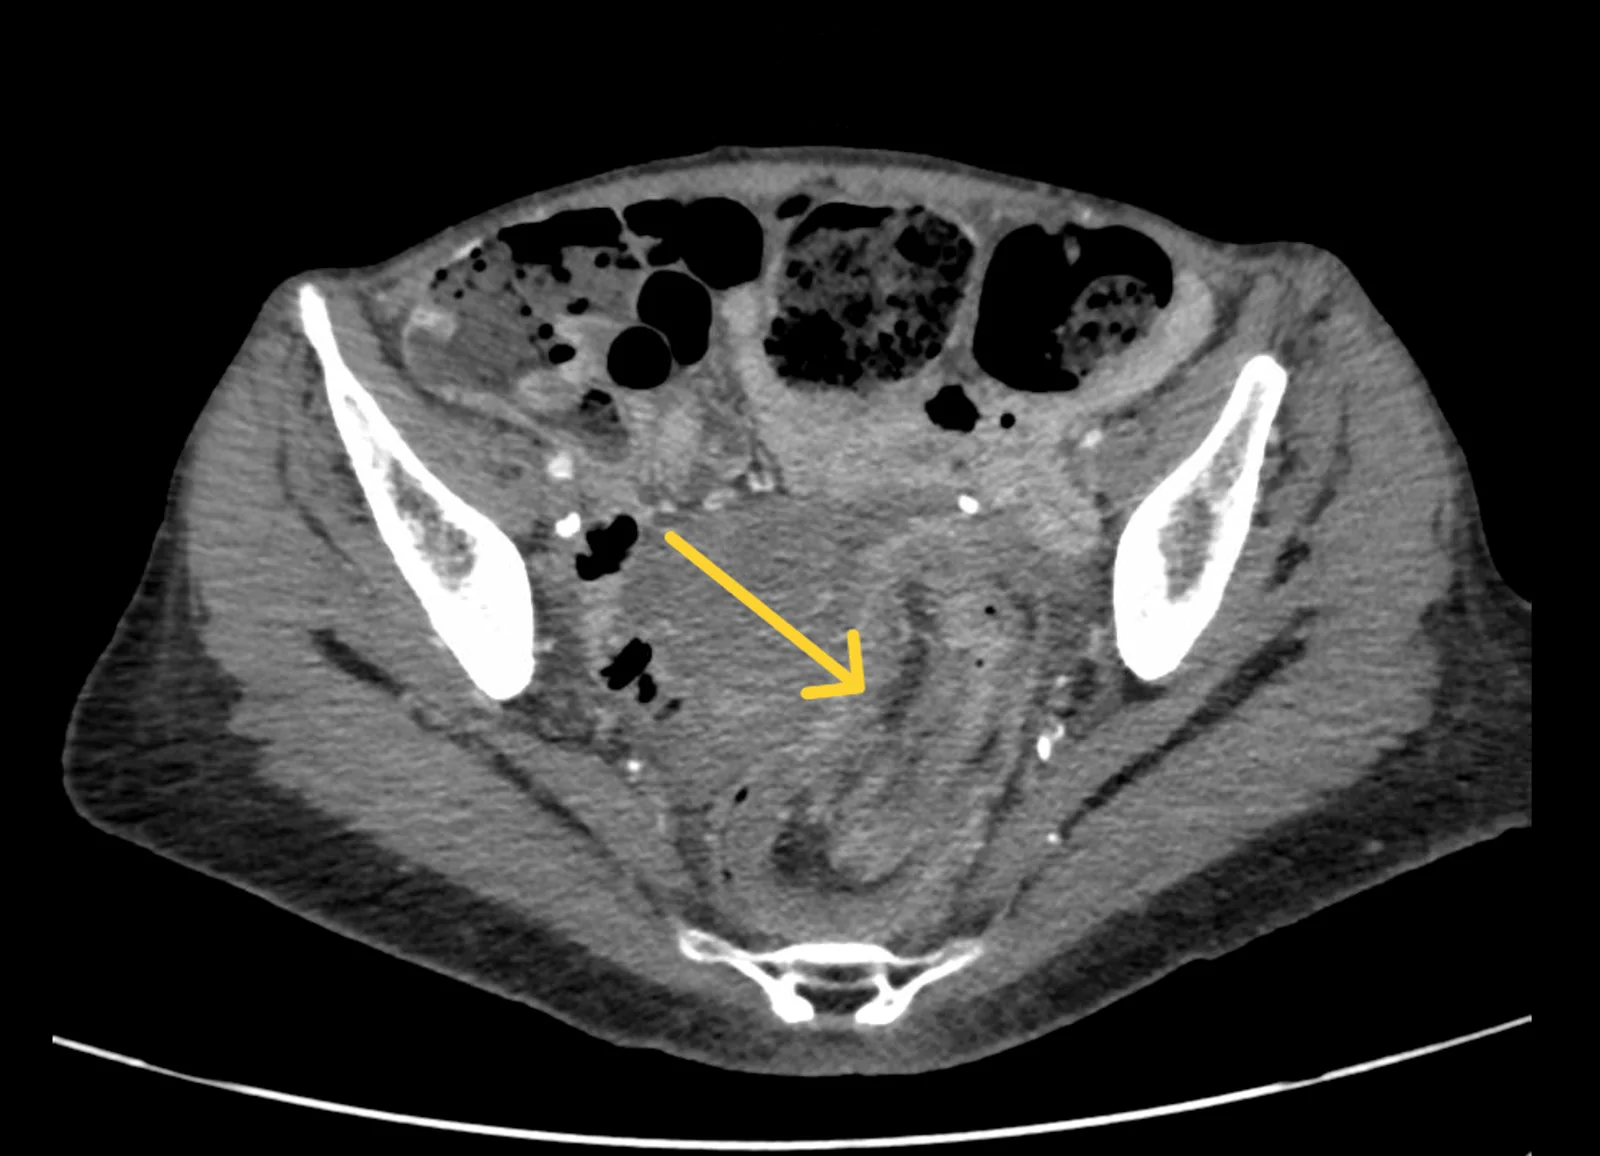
Bowel-in-bowel configuration of rectosigmoid intussusception on CT
